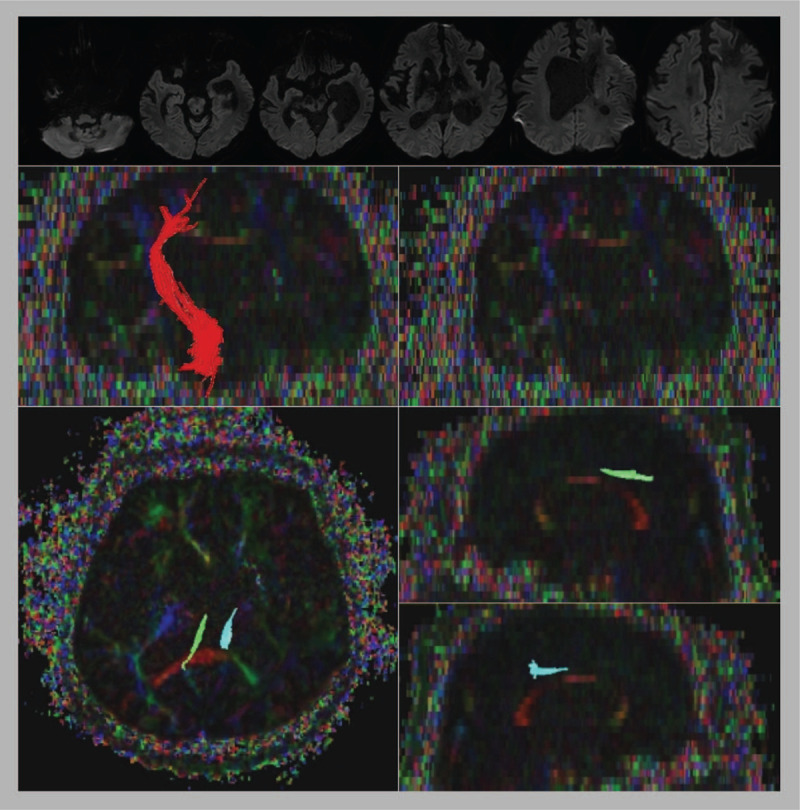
Brain magnetic resonance images and evoked potential studies in patient with PAID. (A) At initial presentation, brain diffusion MRI showed no acute lesions. (B) Tractography of the corticospinal tract was observed only on the left side. In addition, the spinothalamic tract was not detected (C), and disrupted white matters were observed in the cingulum (D–F). The motor evoked potential study, stimulating each hemisphere (upper line, right hemisphere; lower line, left hemisphere) and recording at the first digit interosseous (G) and tibialis anterior muscle (H), showed mild delayed latency and decreased amplitude in the right hemisphere.
